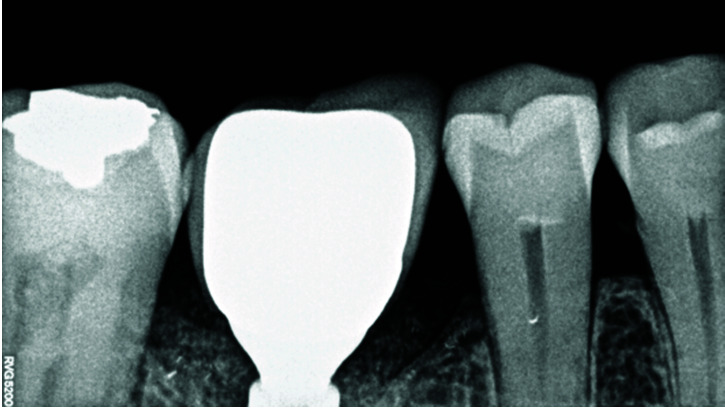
Immediate post-op radiograph demonstrating the grafted material in the defect
